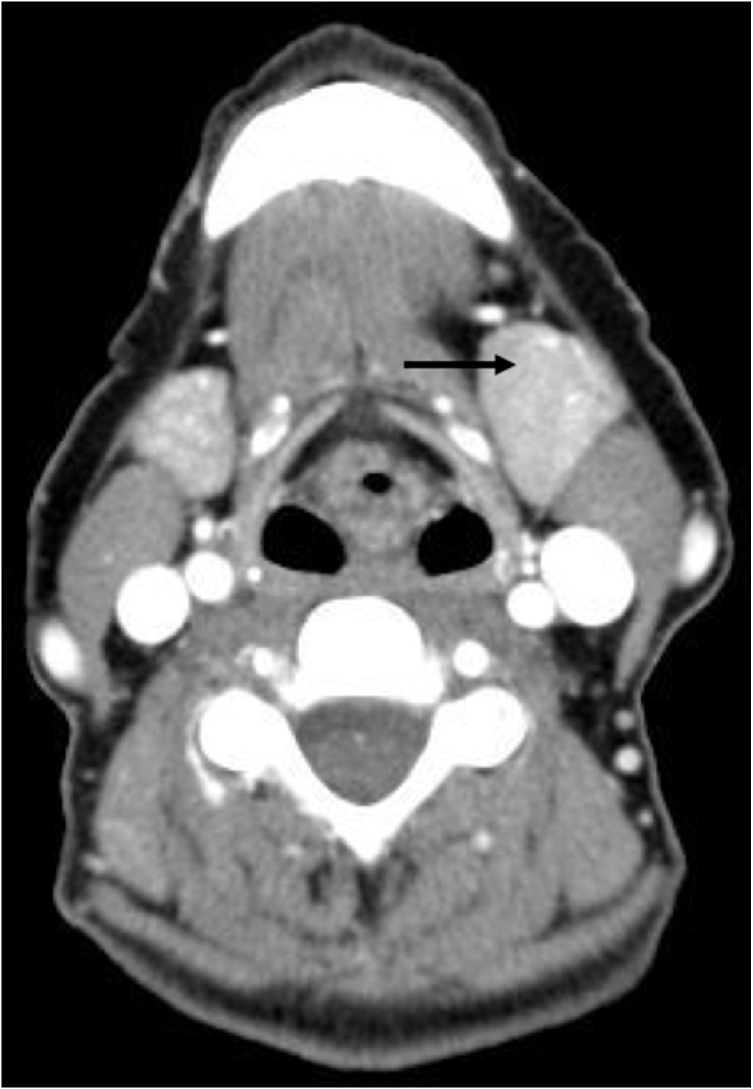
Computed tomography image of the patient’s neck demonstrates a homogeneous increase in the size of the left submandibular gland (arrow). No lymphadenopathy was present.
